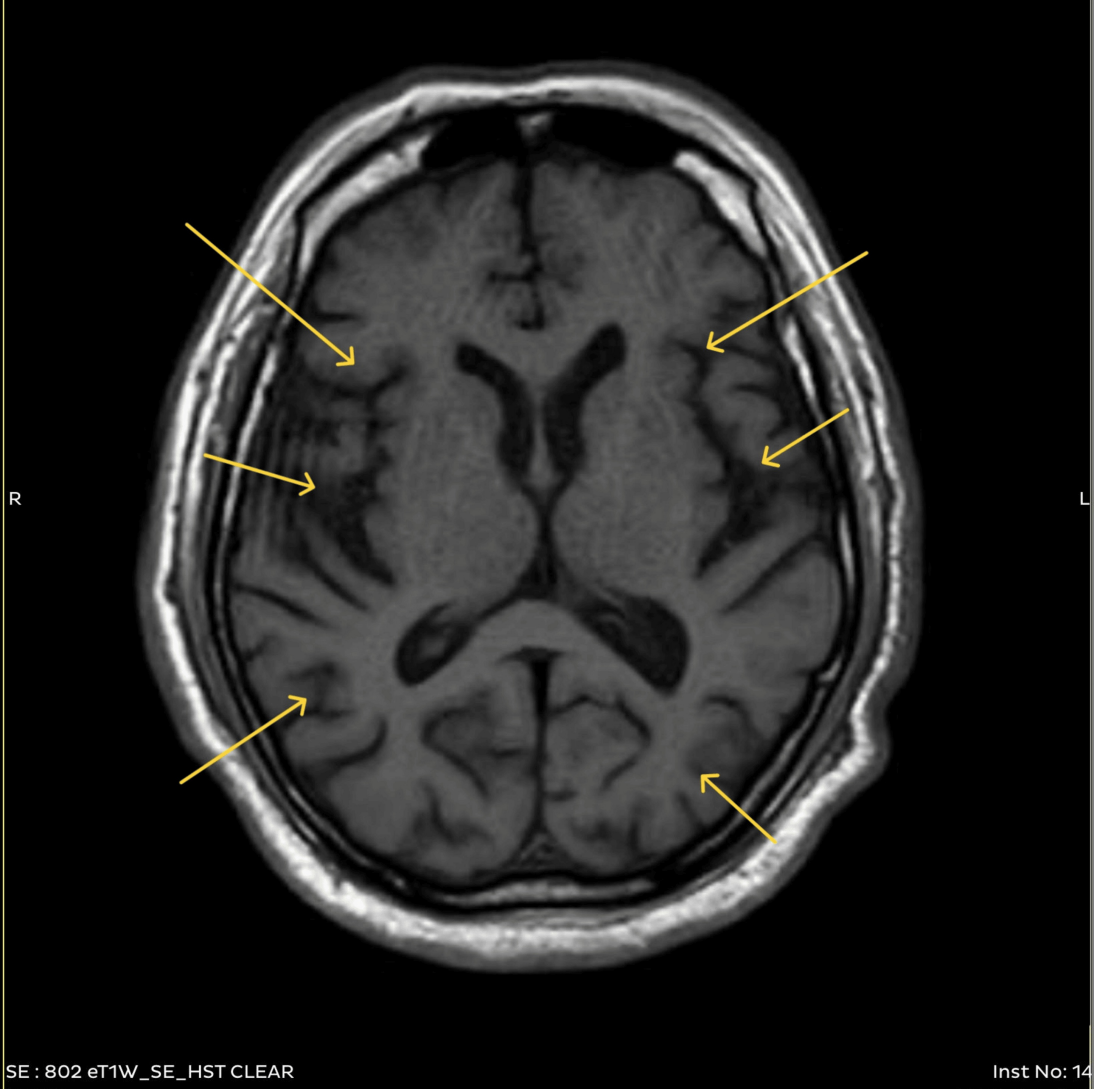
T1W MRI of axial section of the brain showing diffuse brain parenchymal atrophy as evidenced by prominent gyri and sulci T1W MRI: T1-weighted magnetic resonance imaging
